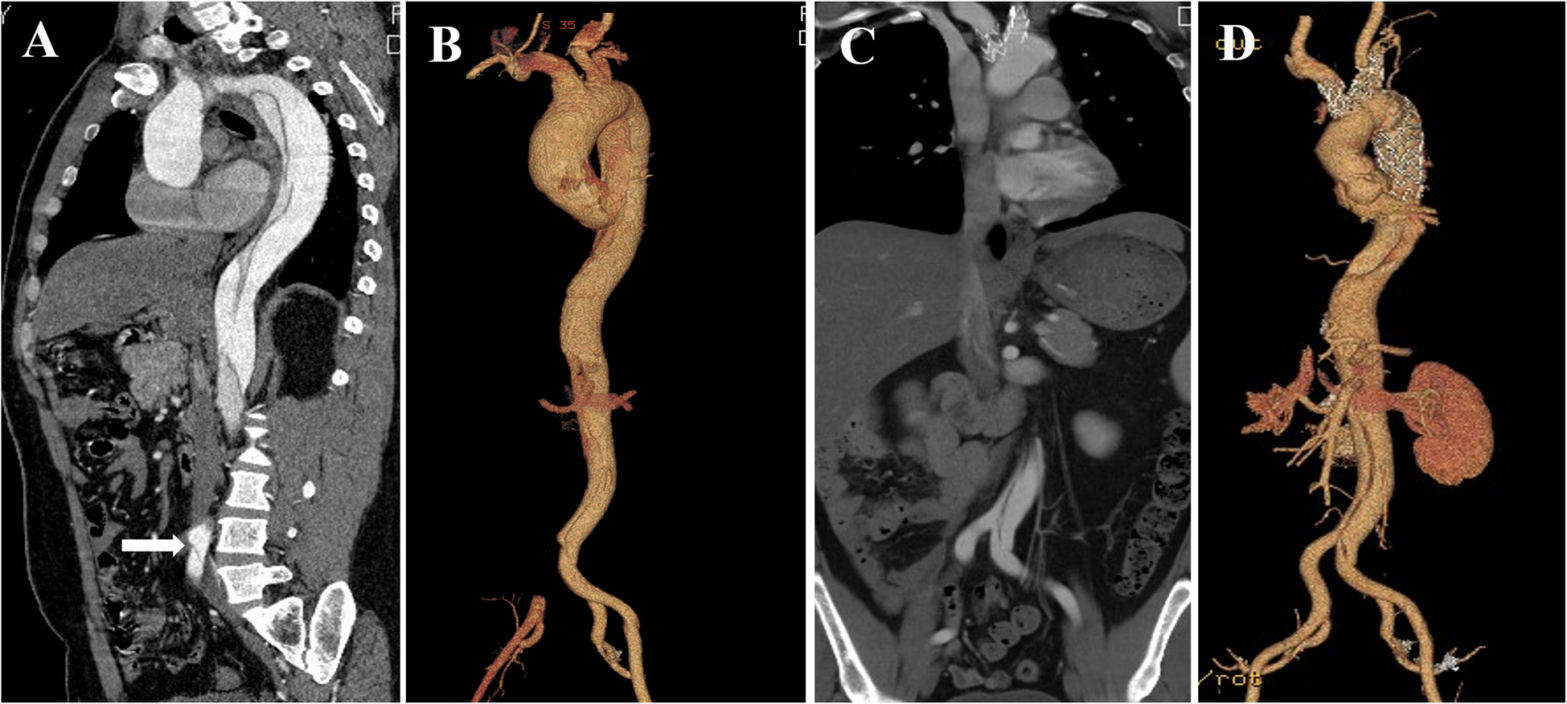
No pulse could be detected from the right lower limb of this patient before the surgery. The skin was colder and paler than another limb. CTs showed an antegrade propagation of the dissection from the ascending aorta to the left iliac artery, accompanied with a complete occlusion of the right common iliac artery, whose orifice was obstructed by a tense false lumen (arrow). Low-density enhanced of the right femoral artery was detected, probably due to the collateral circulation a, b. The patient was performed ascending aorta replacement and triple-branched stent graft implantation. Post-operative CT showed the perfusion of right common iliac artery was complete restored c, d
